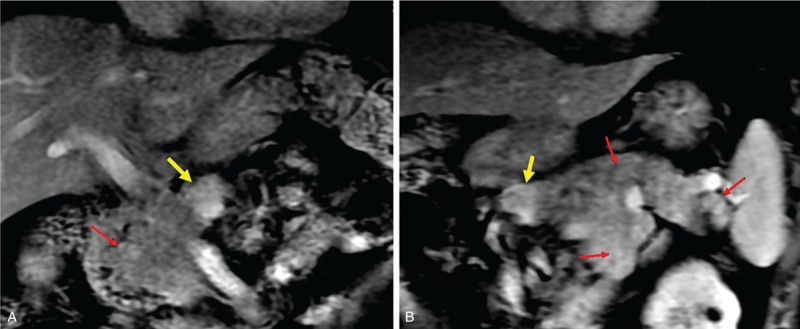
The preoperative magnetic resonance imaging of Case 1 showed multiple tumors in the whole pancreas gland. Yellow arrows: the tumor localized in neck of pancreas; red arrows: the multitumors localized in pancreas.
